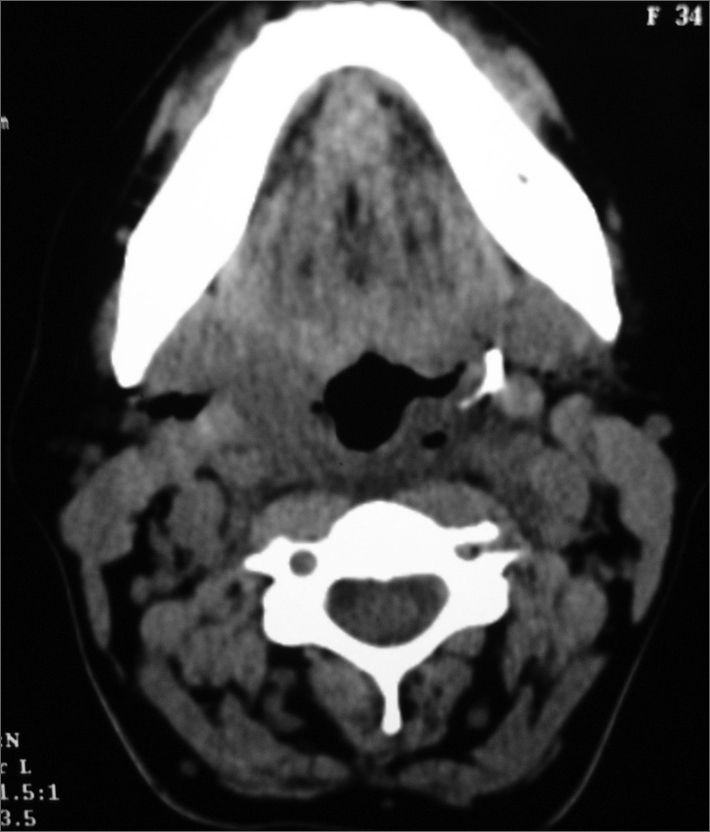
CT scan image showing the foreign body in the left tonsil lodge.
